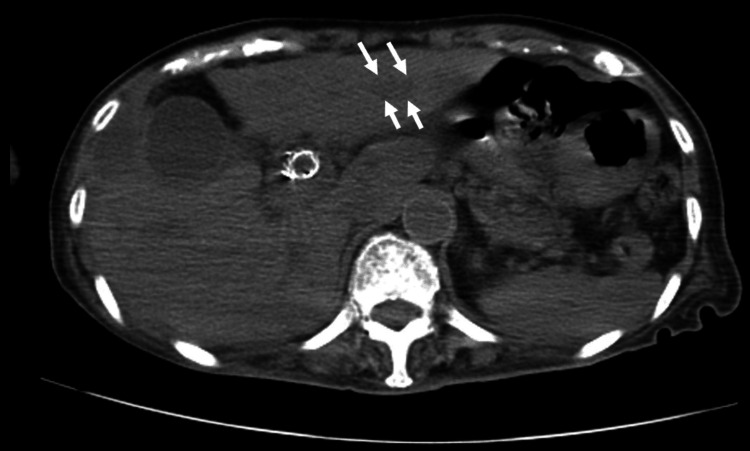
Non-contrast CT at admission Non-contrast abdominal computed tomography (CT) at admission showing the dilatation of the intrahepatic bile ducts (arrow)
